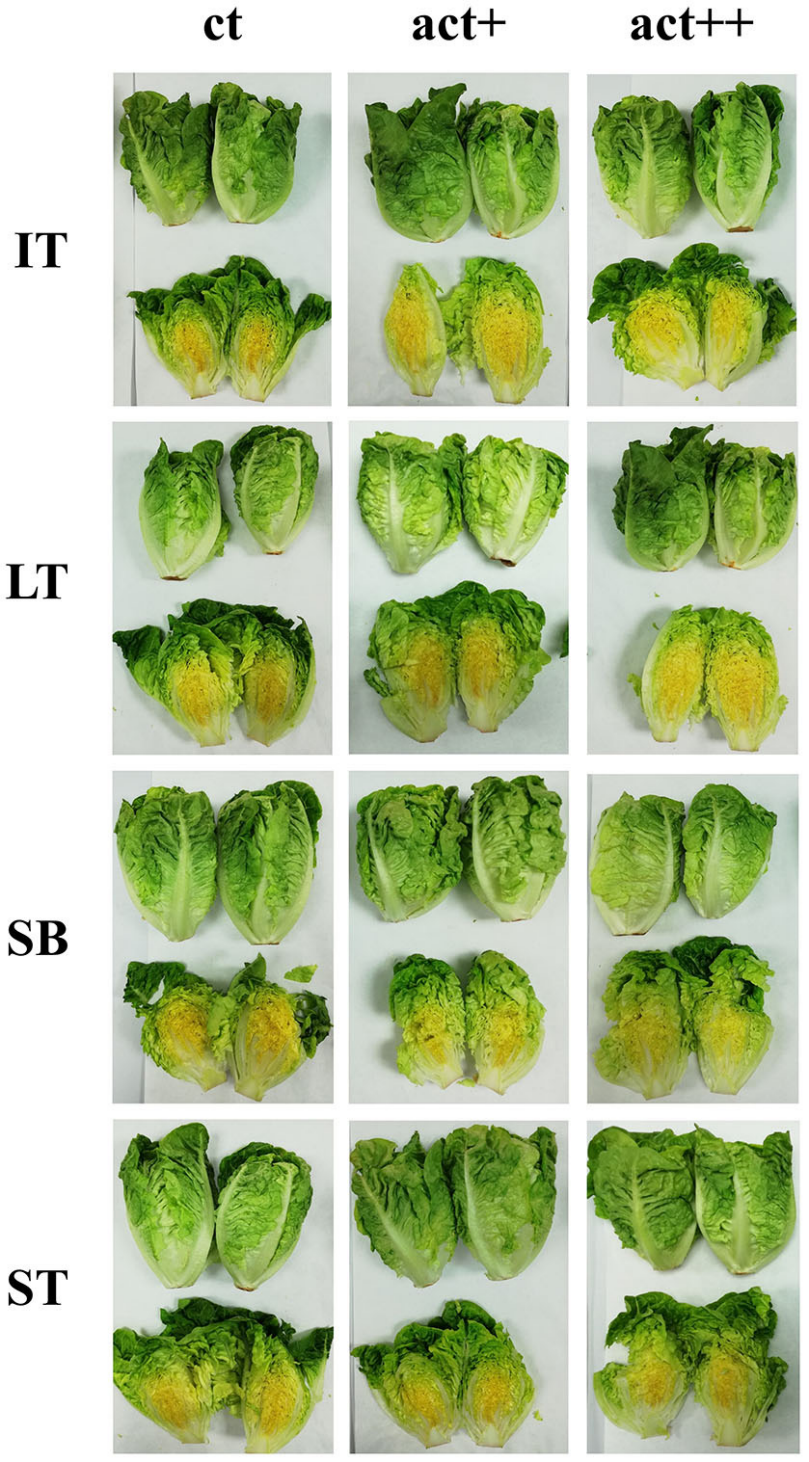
Lettuce stored for 14 days at 2°C packaged within different packaging treatments (industrial tray, IT; large tray, LT; small box, SB; and small tray, ST), either non-active (CT) or active (without cover ^+^; and with cover ^++^).
